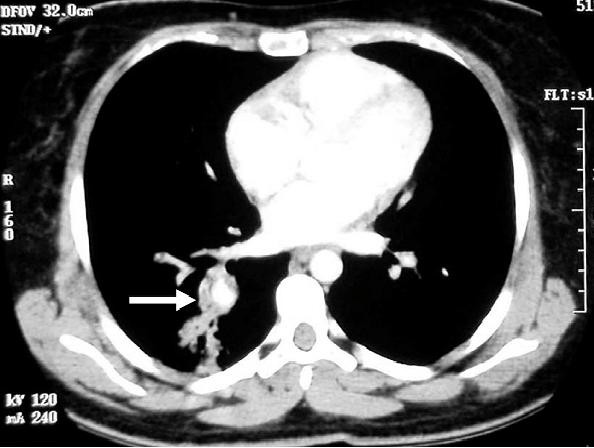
Contrast enhanced CT scan of chest showing pulmonary artery aneurysm. Reproduced with permission from Al-Jahdali H [15].
